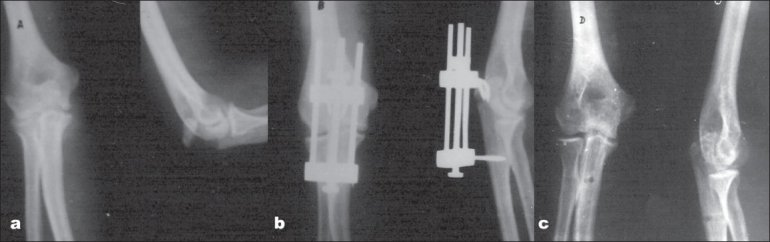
X-rays of the elbow (anteroposterior and lateral views) showing (a) fracture olecranon (b) after clamp application (c) during follow-up after union of fracture and removal of apparatus
